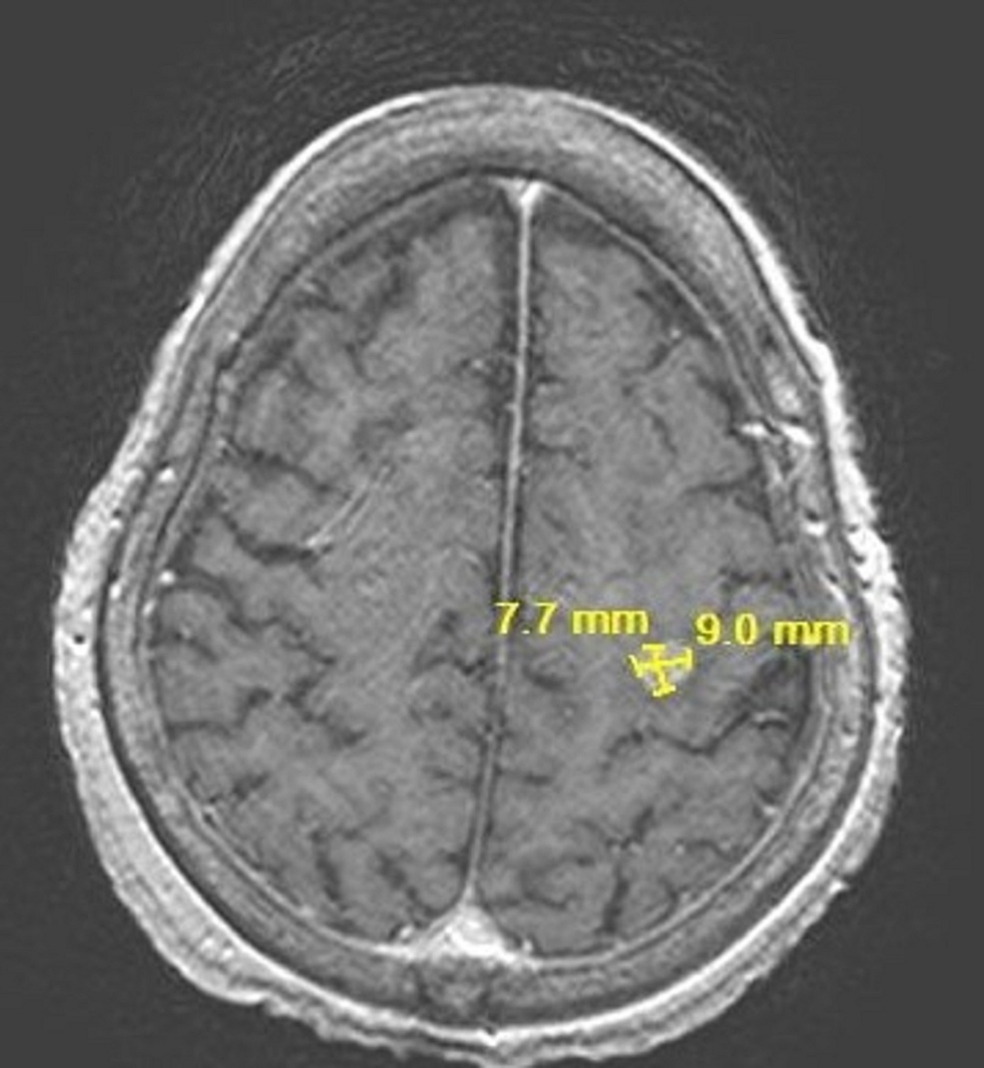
MRI brain showing incidental brain metastatic lesion on 07/08/2021
